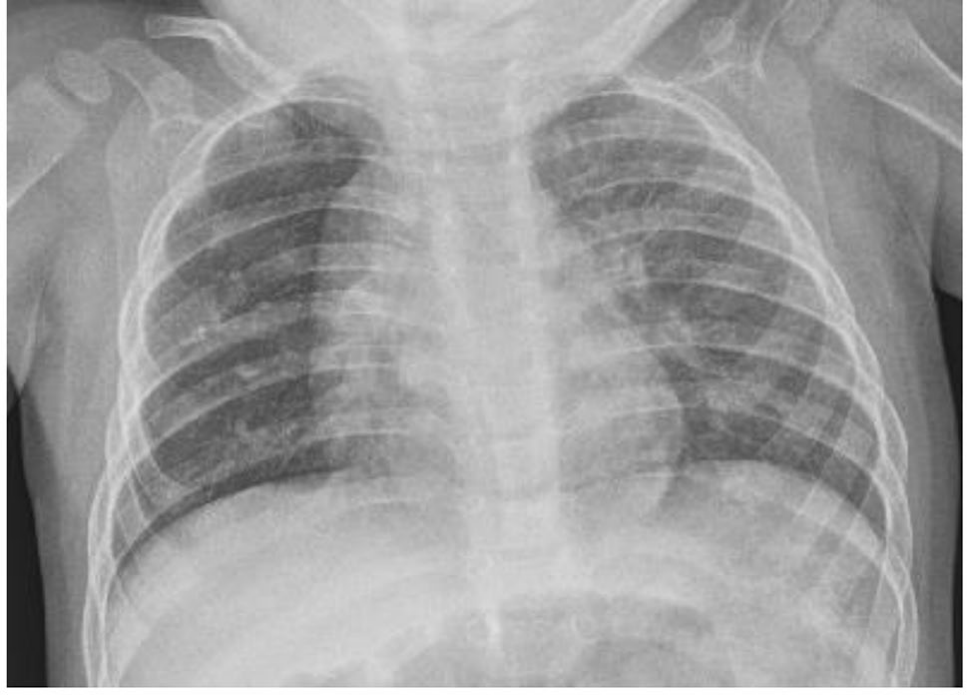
Postoperative Chest X-Ray Demonstrating Right Lung Expansion at 16 Months of Age
